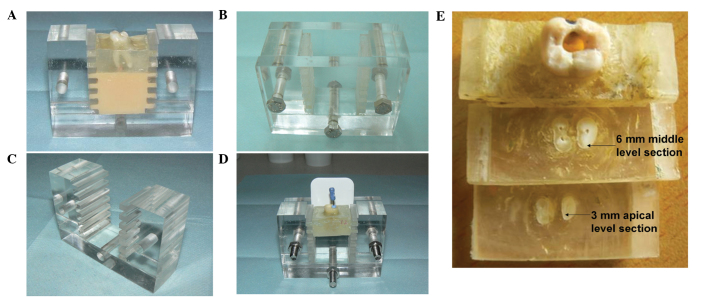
Representative images showing (A) a tooth embedded in clear epoxy resin; (B) the muffle block system; (C) the middle section of the muffle system revealing the grooves; and (D) the file in the canal. Radiographs were obtained in buccolingual and mesiodistal directions. X-ray films were placed behind the teeth for radiography. (E) Two observation levels were selected, one was 3 mm from the apex, defined as the ‘apical level’ and the other was 6 mm from the apex, defined as the ‘middle level’.
